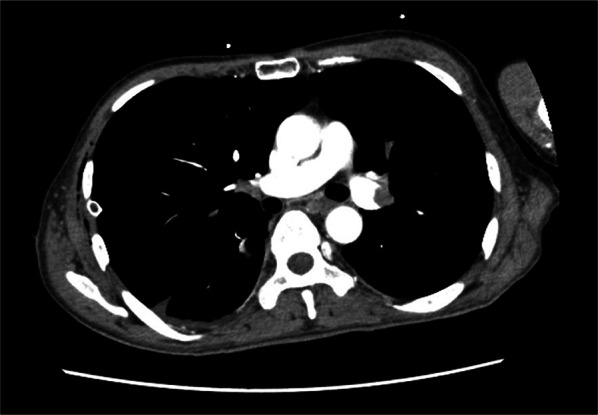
Enhanced CT scan findings after sudden dyspnea and dropped SpO_2_. The enhanced CT scan showed Pulmonary thromboembolism due to thrombus in the left main pulmonary artery
